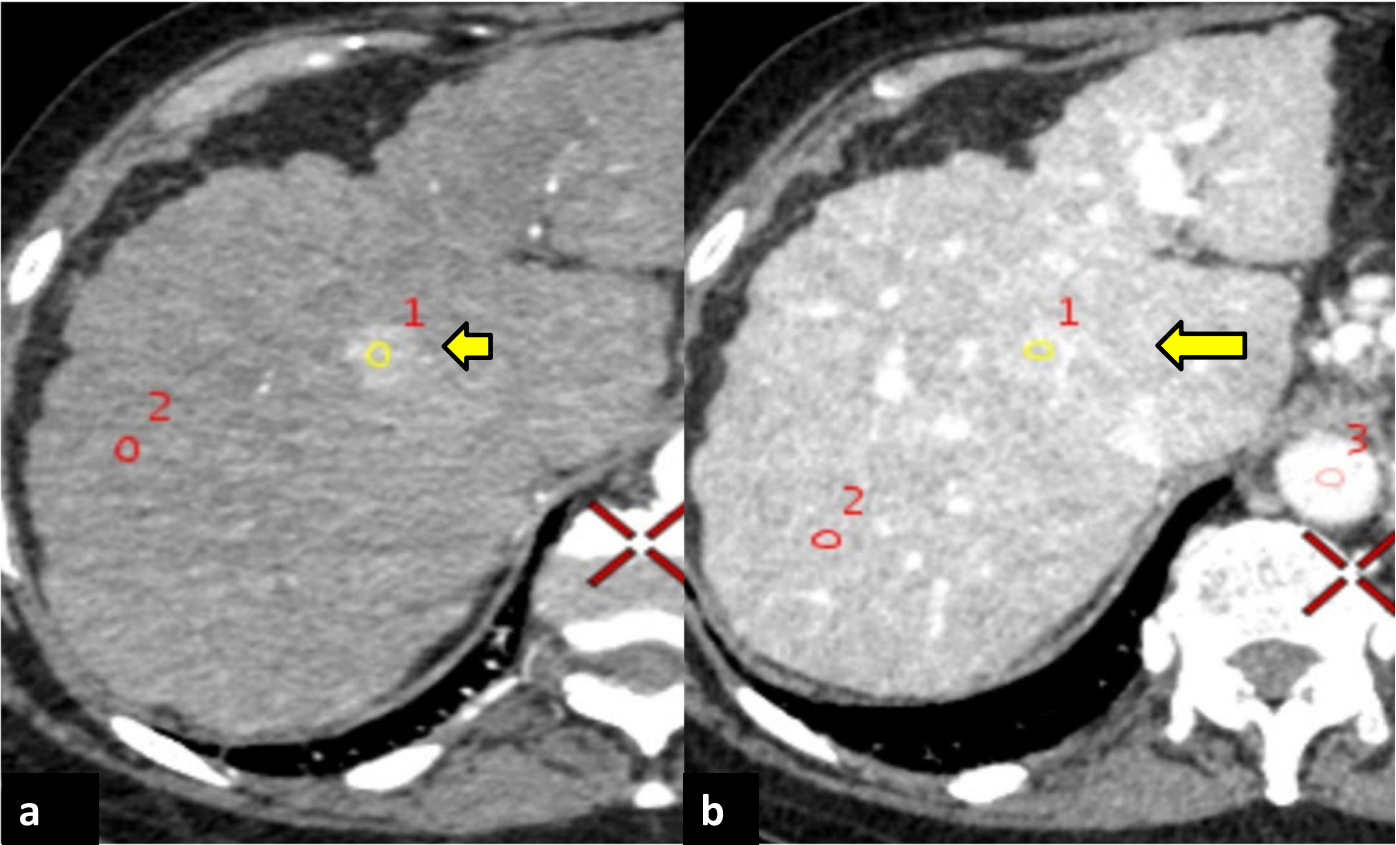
Computed tomography of a patient with underlying cirrhosis depicting an ‘indeterminate’ nodule. a Polychromatic axial image on HAP depicting a hypervascular nodule (yellow ROI), in segment VIII (yellow arrow). b Polychromatic axial image on PVP of the same nodule shows persistent enhancement without overt washout (yellow ROI) in segment VIII (yellow arrow)
